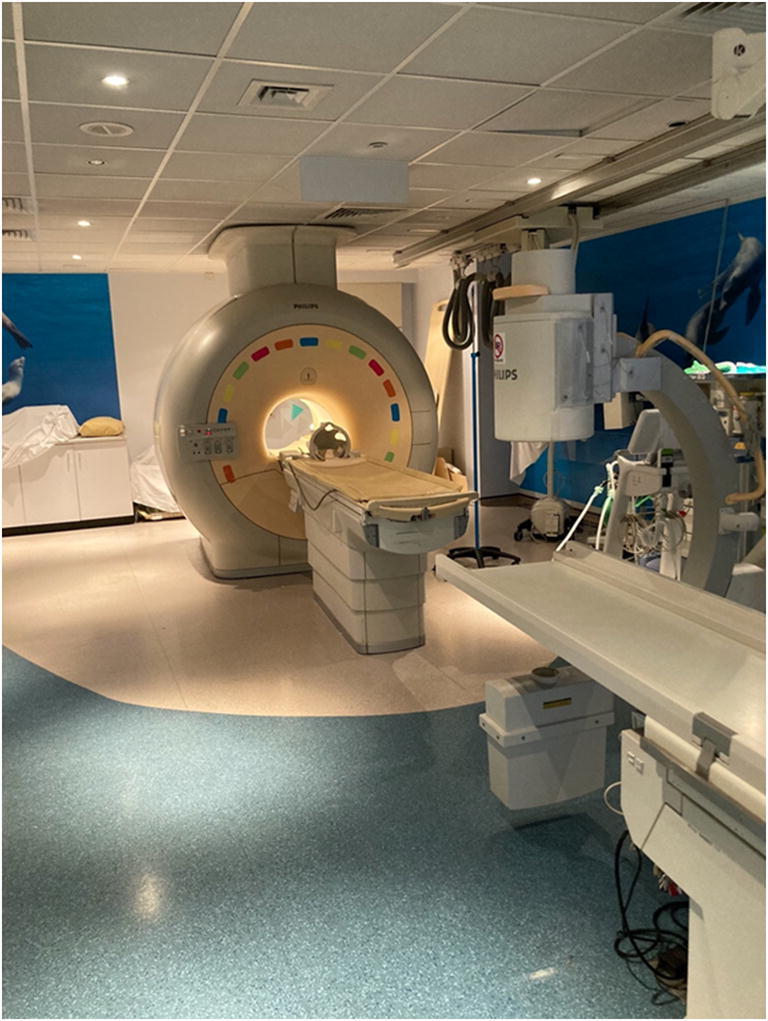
X-ray and CMR (XMR) room. XMR room with the x-ray and MR equipment joined by a movable tabletop. The c-arm of the x-ray unit is seen in the right side and the 5-gauss area is demarcated by a change in the floor colouring from the MR to the x-ray end of the room
